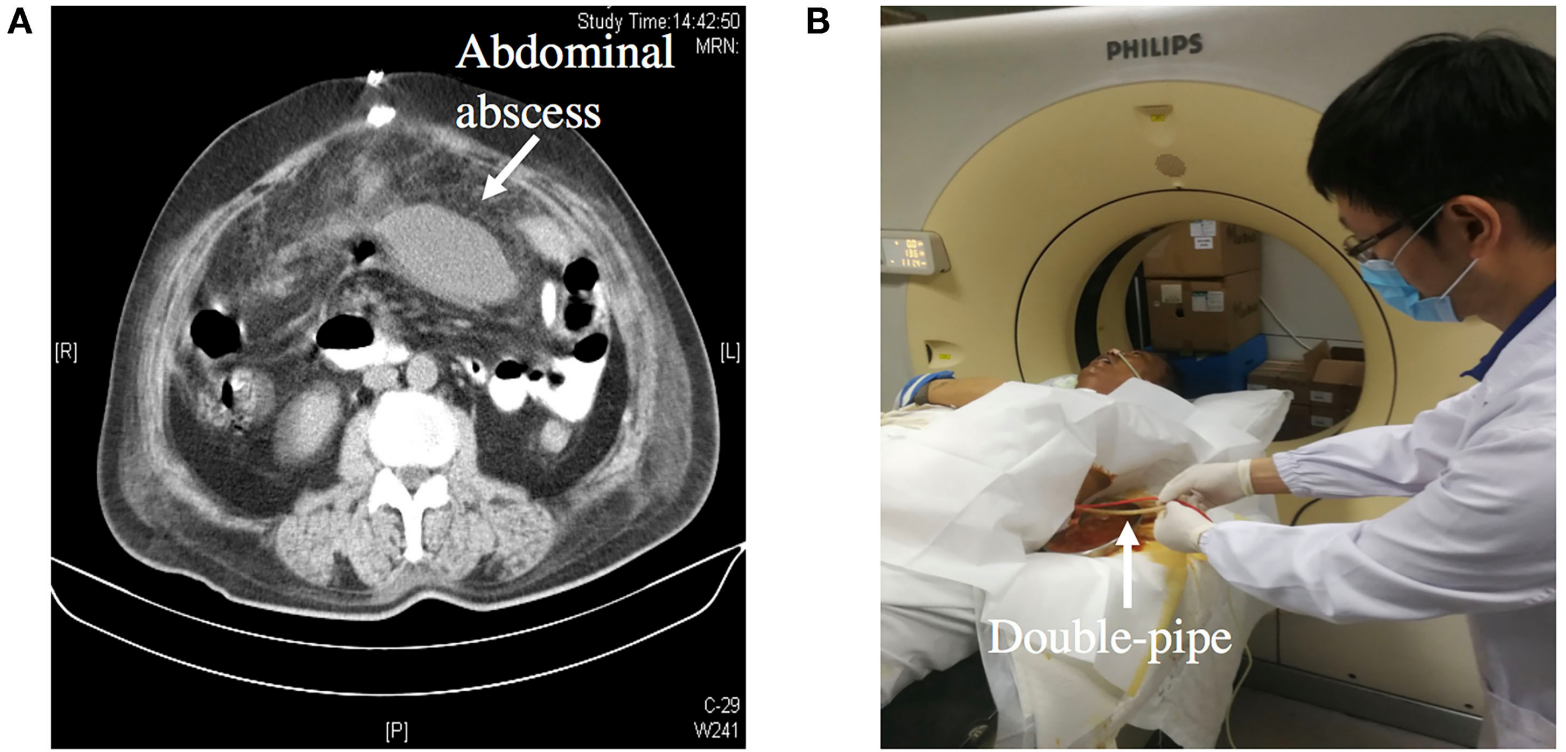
(A) Showing an abdominal abscess due to ECF. (B) Showing CT-guided double-pipe placement for continuous irrigation and drainage of the abscess.
